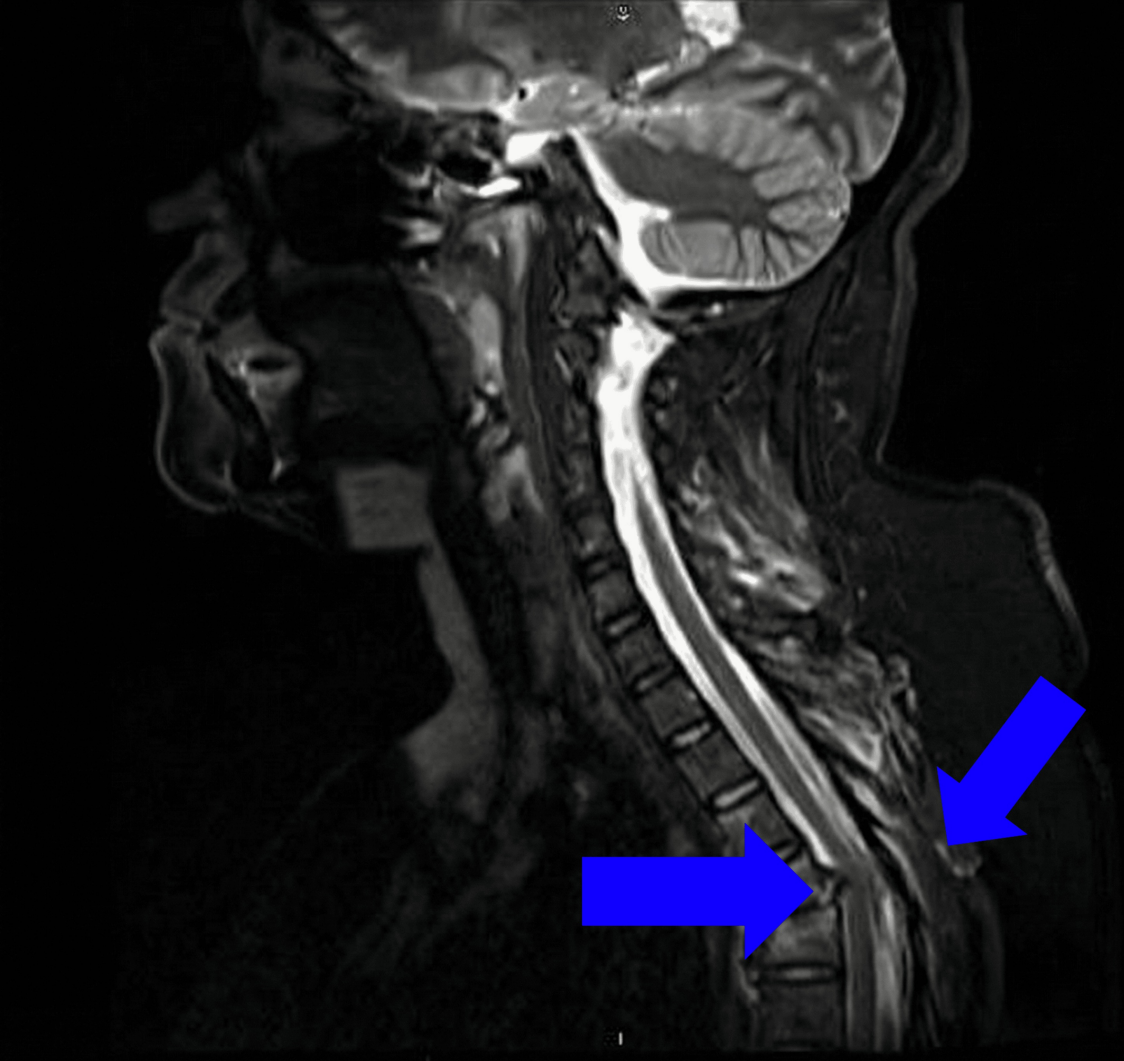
Sagittal MRI demonstrating the spinal cord MRI showed absence/agenesis of the posterior ligamentous complex, with no evidence of spinal cord compression, edema, or intramedullary hyperintensity.
